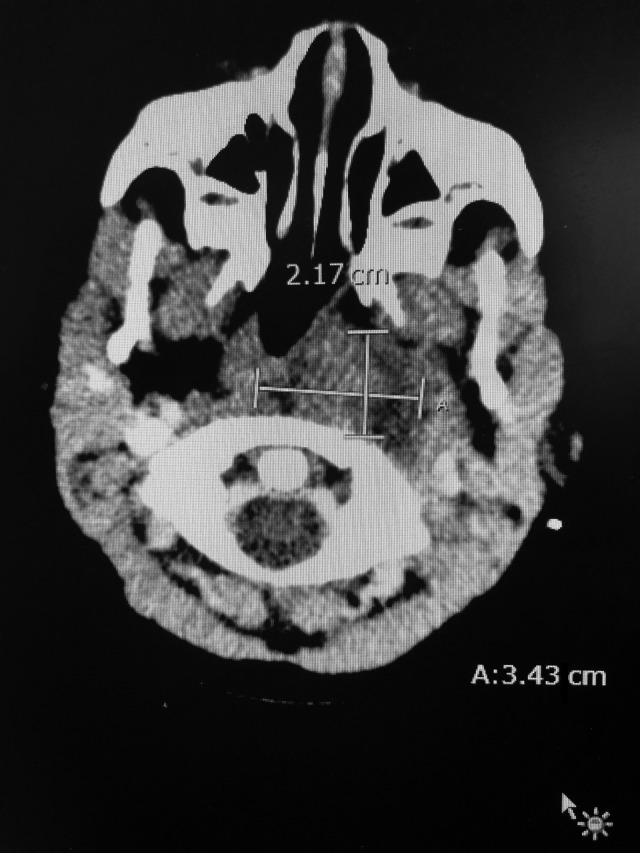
Section of the CT shows the fluid present in the nasopharyngeal region is 3.4 cm transverse by 2.2 cm AP
